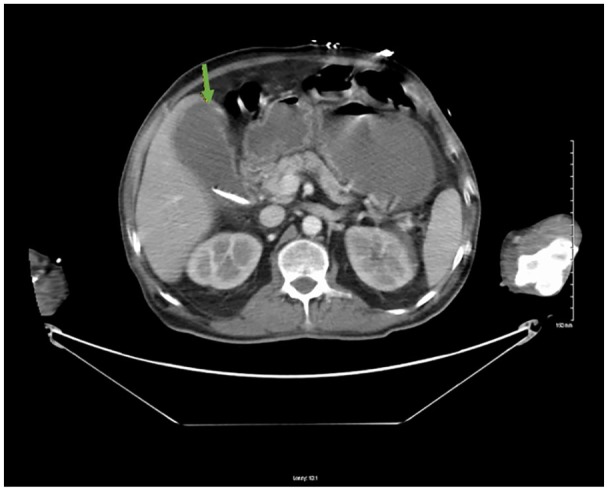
Computed tomography of the abdomen with contrast demonstrating mildly
